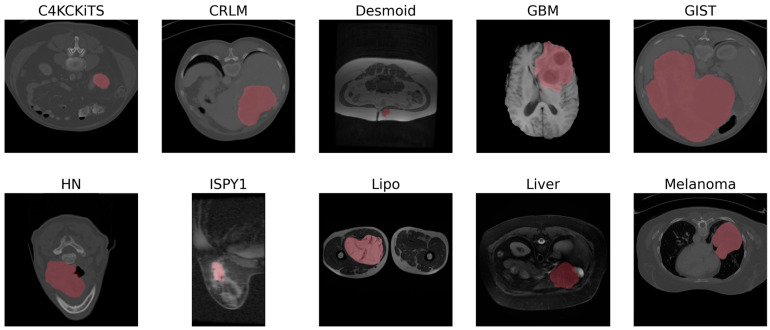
Example slices for each dataset. Images were normalized to the range between 0 and 255, regardless of whether they were CT or MR sequences. The segmentation is depicted in red. The HN image was cropped for visualization purposes.
